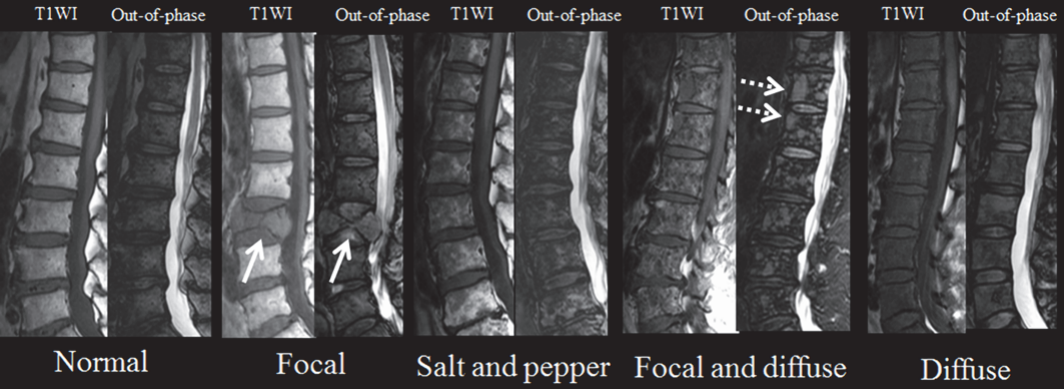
MRI of the lumbar spine shows infiltration patterns of multiple myeloma. In the focal pattern, focal areas of decreased signal intensity are seen on T1-weighted imaging. Out-of-phase image shows increased signal intensity in the corresponding areas (white arrows). In the salt-and-pepper pattern, T1-weighted imaging shows intermingled hyper- and hypointense signals. Areas of signal hyperintensity on T1-weighted imaging correspond to signal-hyperintense areas on out-of-phase images. In the focal and diffuse patterns, T1-weighted imaging shows diffusely decreased signal intensity of vertebrae and focal areas of signal hypointensity are also seen (white dotted arrows). T1WI, T1-weighted imaging.
